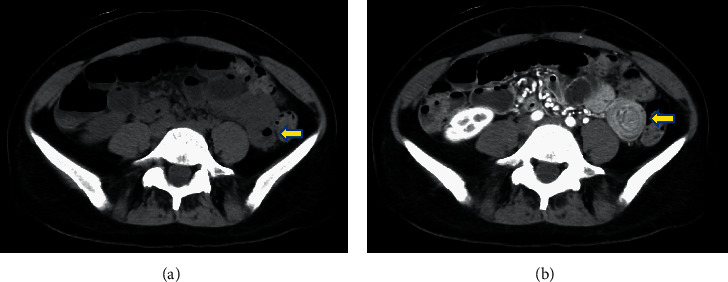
(a) Computed tomography (CT) without contract was largely unremarkable, with no visible signs of tumor or bowel obstruction in the jejunum (arrow). (b) CT with contract taken several minutes later revealed a bowel-in-bowel configuration with invaginated mesentery, consistent with jejunojejunal intussusception (arrow). No mass was visualized.
